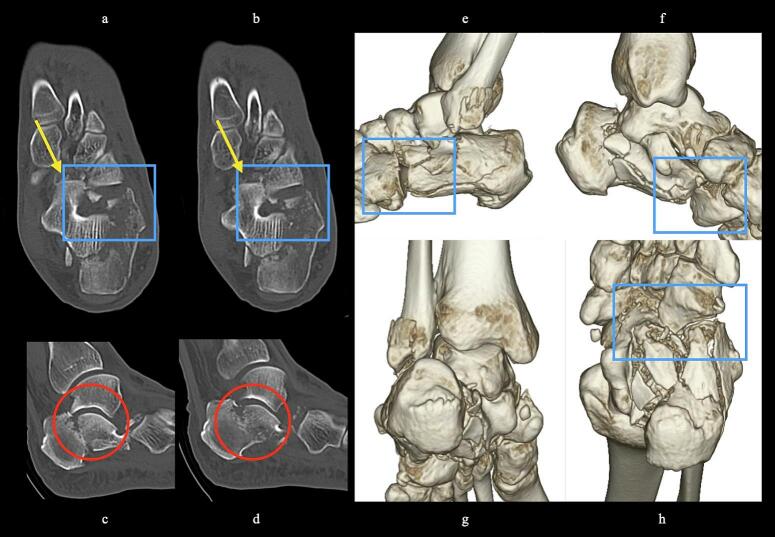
CT at the time of injury. a-b:Horizontal view. Dislocation of the calcaneocuboid joint is present(yellow arrow). Comminuted and depressed fracture of the calcaneocuboid articular surface of the calcaneus(blue box) with marked bulging of the lateral wall. c-d:Siggital view. No comminuting of the posterior talocalcaneal articular surface, but significant depression and the depressed bone fragments are in contact with the basolateral cortex(red circle). Shortening and significant decreesing in calcaneal height are also present. e-h: 3DCT Strong comminuted of the lateral wall and anterior basolateral side, lateral deviation and shortening of the calcaneal tubercle and bulging of the medial and lateral wall are also significant. (For interpretation of the references to colour in this figure legend, the reader is referred to the web version of this article.)
